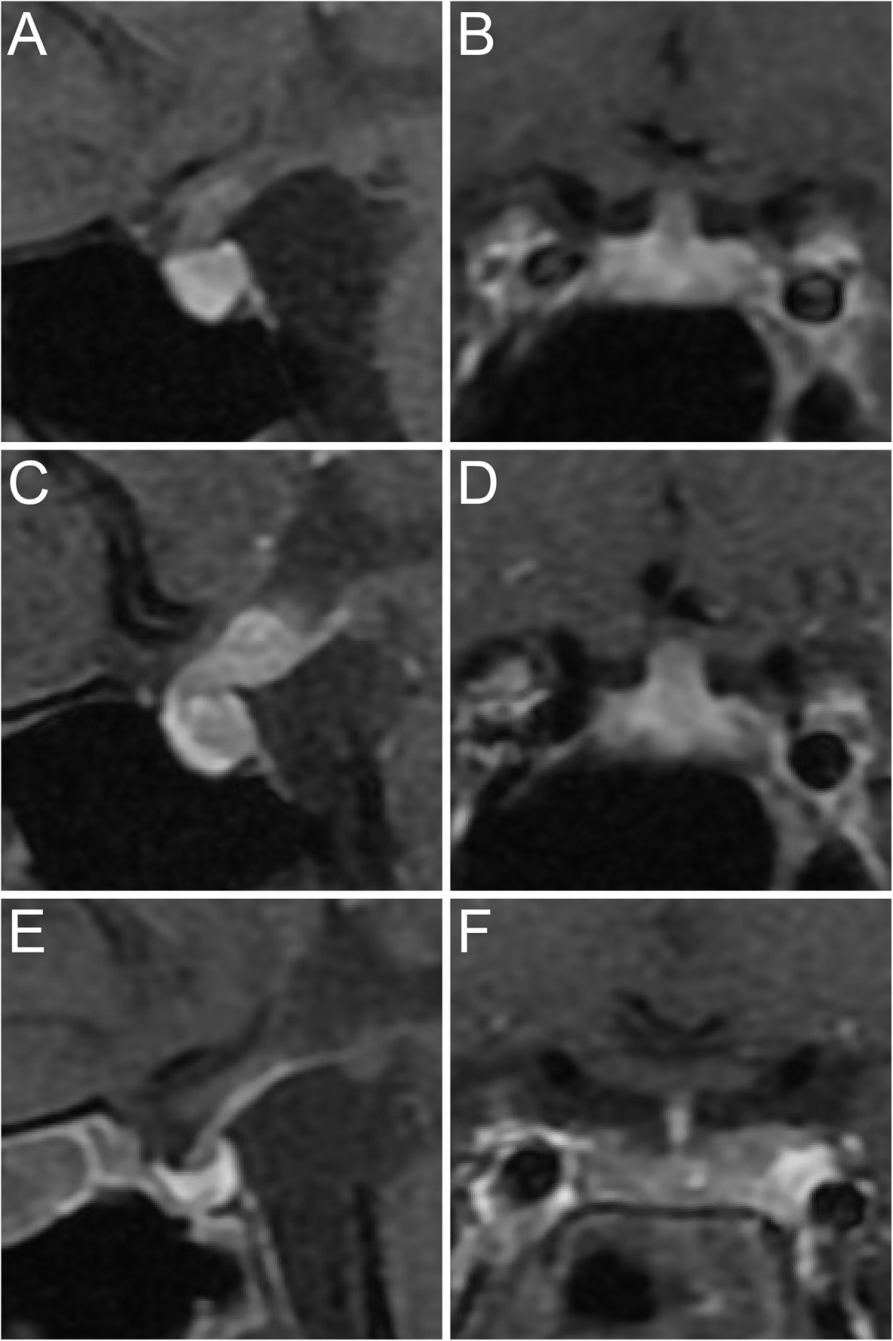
Contrast enhanced MRI of the pituitary. (A, B) Sellar mass and pituitary stalk thickening before treatment. (C, D) The sellar and stalk lesion progressed after treatment by prednisone and mycophenolate mofetil. (E, F) The sellar and stalk lesion shrank after four cycles of chemotherapy
